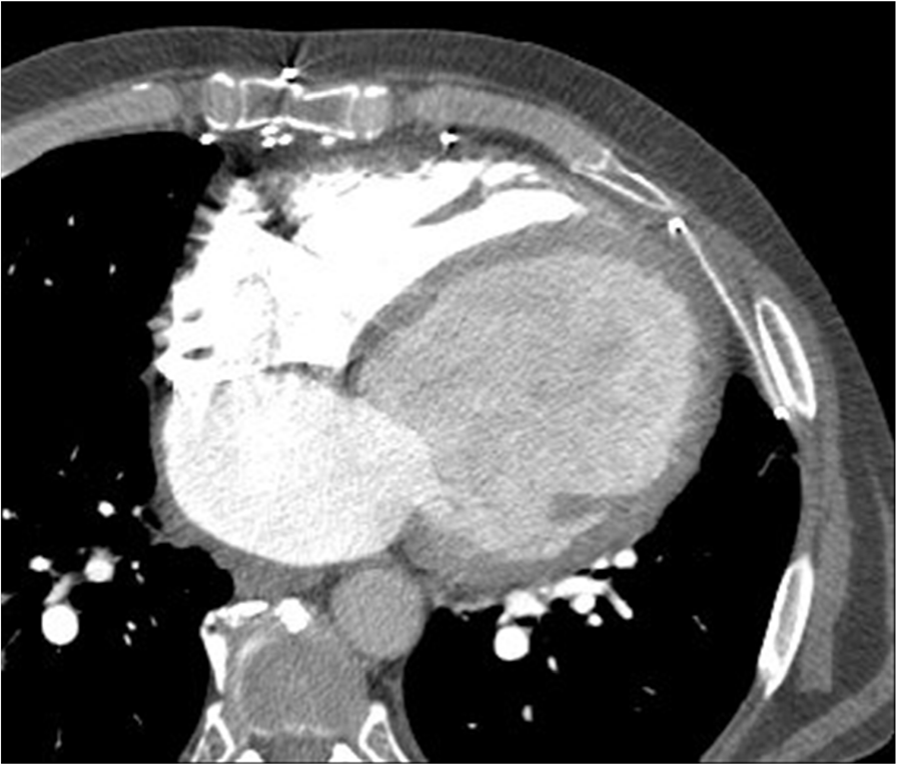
Axial contrast-enhanced CT demonstrates dilated cardiomyopathy (DCM) on an axial contrast enhanced image in a 65-year-old man being evaluated for PE
